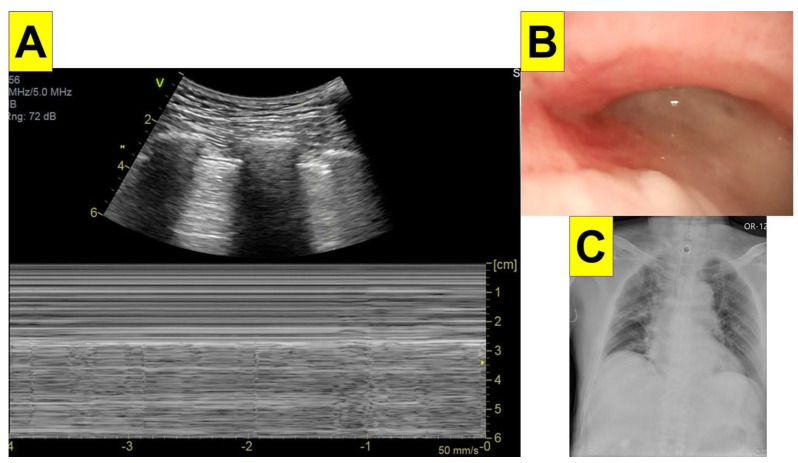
Clinical findings of acute hypoxemia during anesthesia. (A) Lung POCUS image; (B) bronchoscopic findings via tracheostomy tube; (C) portable chest radiograph taken shortly after the incident.
